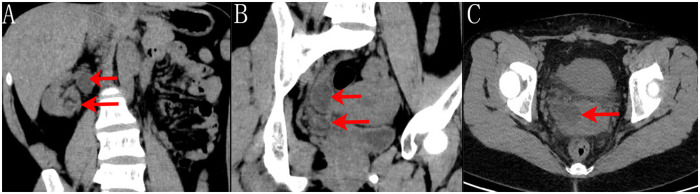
Unenhanced CT of the urinary tract. (A,B) Oblique coronal reformatted images demonstrate a duplex kidney with ureteral duplication. The upper pole moiety and corresponding ureter are dilated (short arrows), while the lower pole moiety and ureter are normal (long arrows). (C) Axial image shows the dilated distal ureter adjacent to the vagina.
